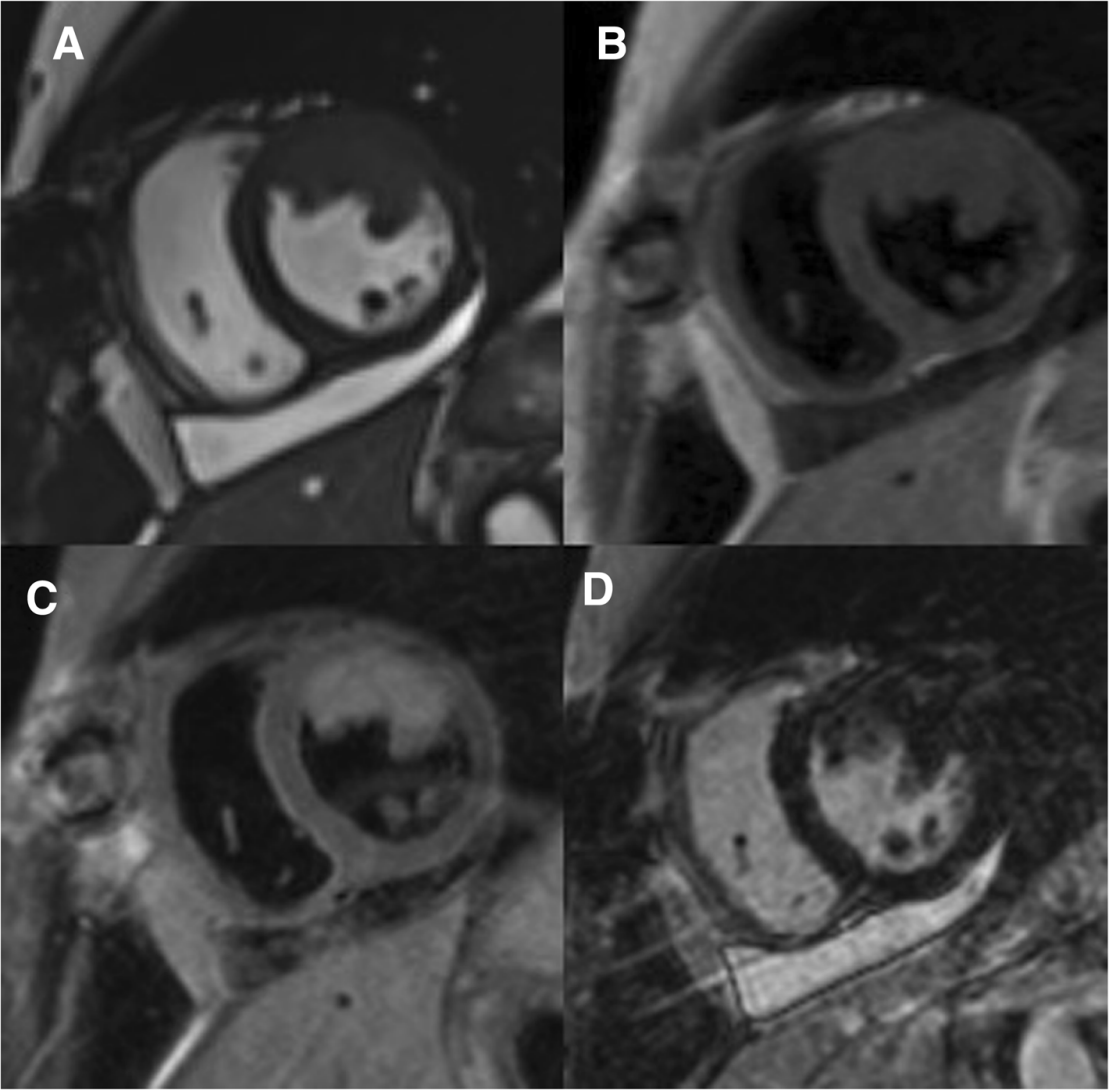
Residual tumor masses after cytoreductive surgery in the anterior and lateral wall of LV in cine (a), T1-weighted (b), T2-weighted (c) and LGE (d) images
